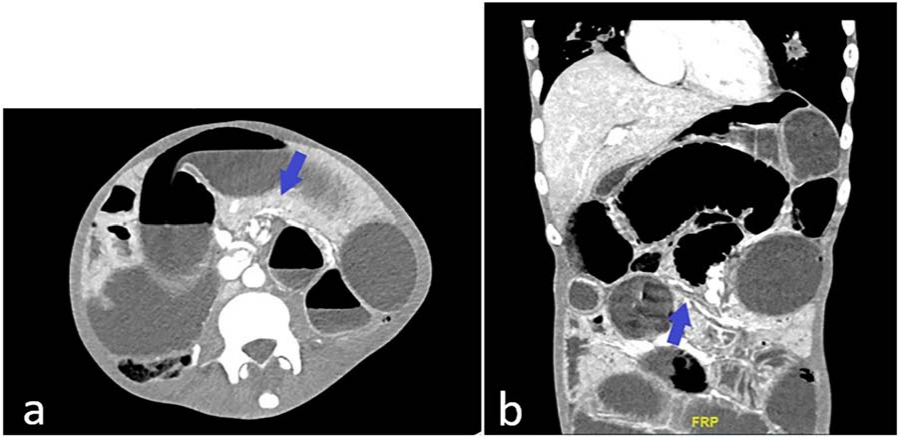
(a) CT abdomen and pelvis showing possible swirling of mesentery (blue arrow); (b) CT abdomen and pelvis showing dilated and collapsed small bowel loops suggestive of transition zone (blue arrow).
